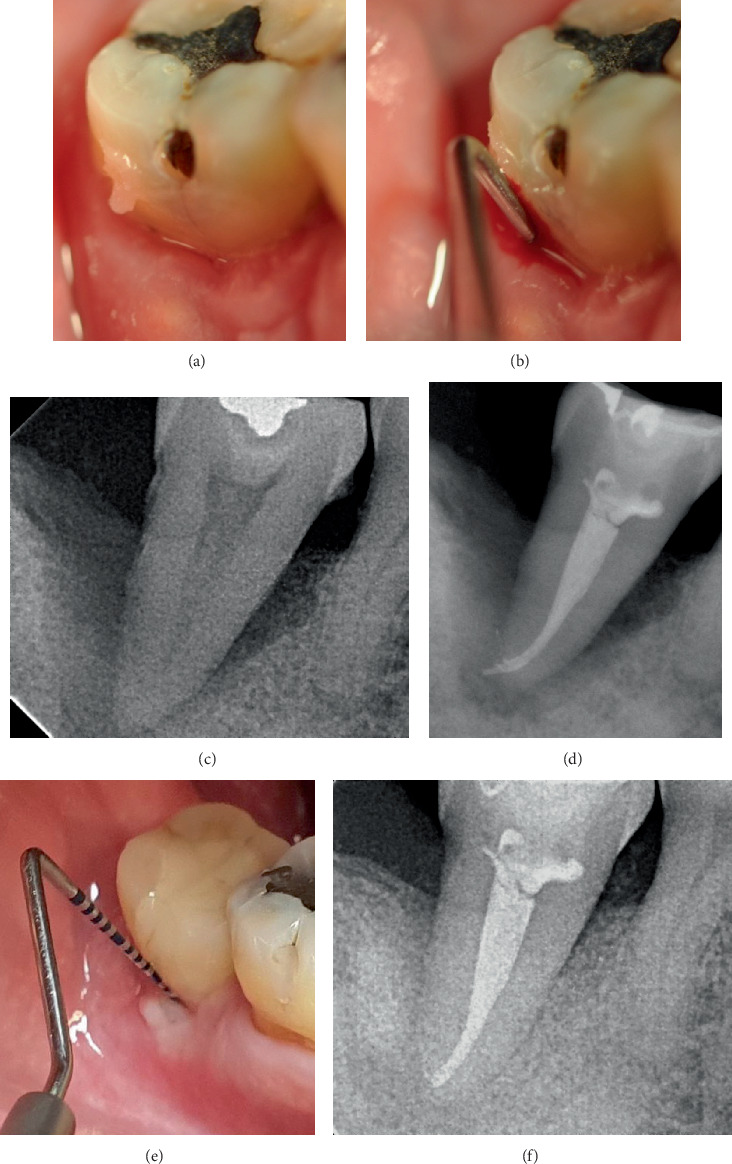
Clinical presentation of Case 2. (a) Presurgical clinical view. (b) A 15-mm periodontal pocket depth on the buccal aspect of Tooth #47. (c) Preoperative radiographic view showing severe bone loss extending to the apex of Tooth #47. (d) Root canal was performed before periodontal regeneration surgery. (e) Postoperative views at 5 years of follow-up. Probing depth was reduced to 2 mm. (f) After 5 years, an improvement in the intrabony component of the defect can be observed.
